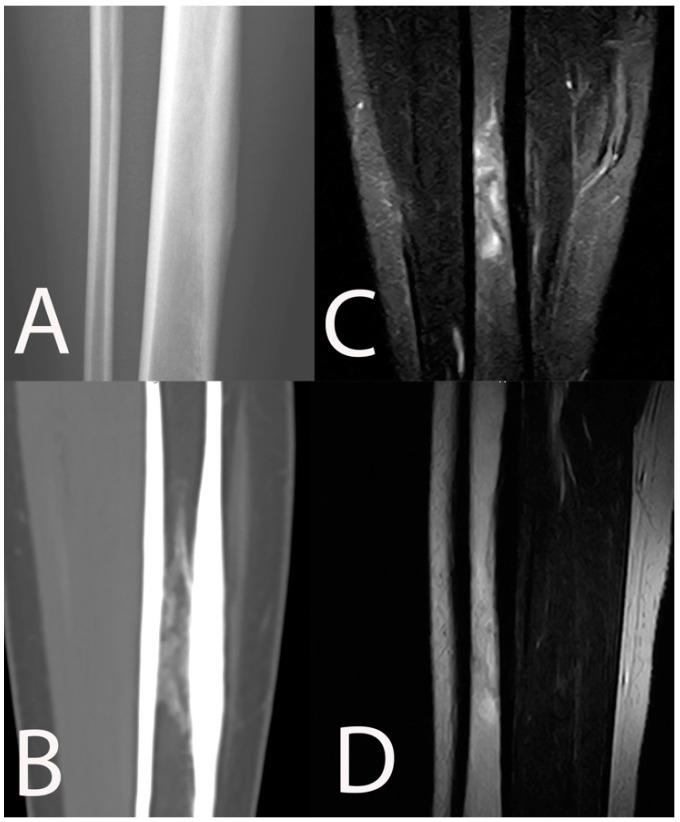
Chronic osteomyelitis of the tibia in a 16-year-old female. Periosteal reaction and sclerotic intramedullary focus are detectable on conventional radiography (A) and CT scan (B). MRI showed ill-defined bone edema among the sclerotic intramedullary changes on STIR coronal (C) and T1w sagittal (D).
